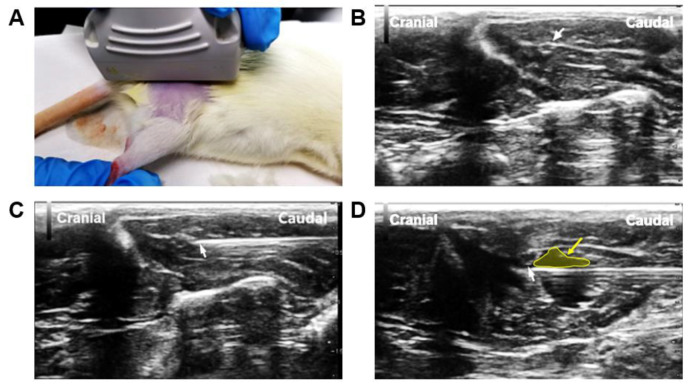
Demonstration of local lidocaine injection under the guidance of an ultrasound. (A) The position of the ultrasonic probe placement (L55/5–13 MHz, Hitachi noblus, Japan). (B) Ultrasound image of the severed sciatic nerve (the white arrow indicated the proximal end of the nerve). (C) Ultrasound-guided injection (the white arrow indicated the tip of the needle). (D) Image after lidocaine/saline injection (white arrow indicated tip of the needle; the yellow arrow and area indicated injected fluid).
